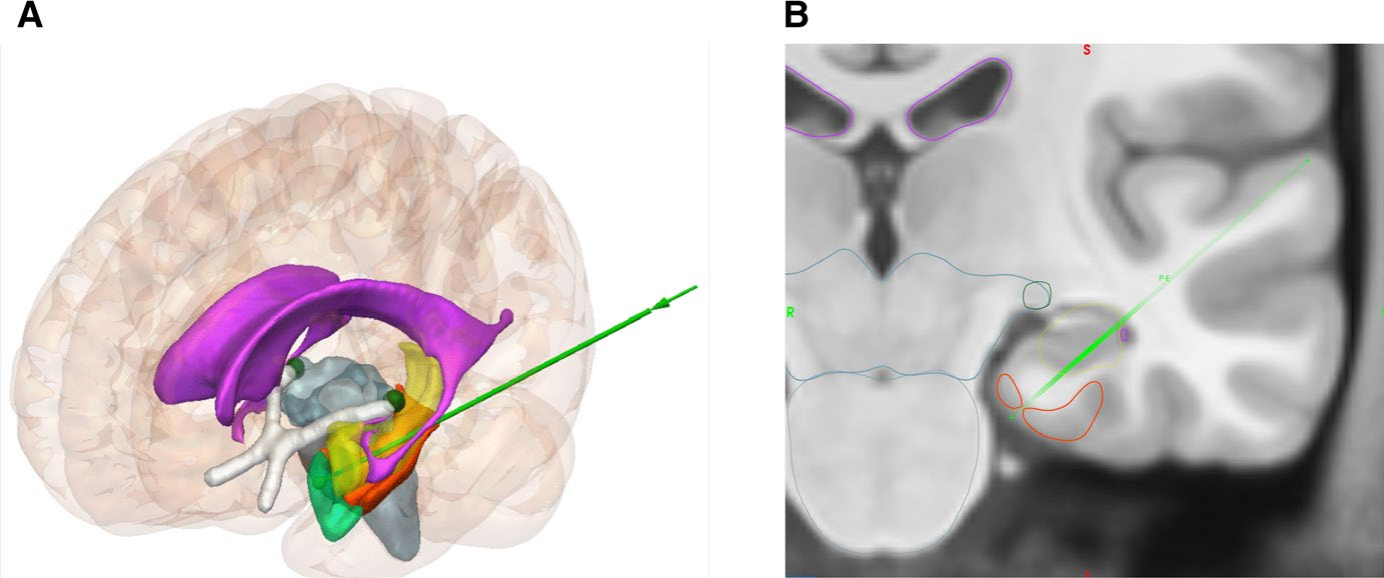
Template‐based A, 3D model and B, coronal MRI of an automated left sided LITT trajectory (light green) at the level of the LGN (dark green) showing its incorporation within the brainstem segmentation (blue). Entire LITT trajectory overlaid onto 2D coronal slice with intrahippocampal (opaque green) and extrahippocampal (transparent green) portions delineating the lateral to medial and superior to inferior angulation of the trajectory. Position of trajectory at level of LGN (green). Amygdalohippocampal complex (yellow), lateral ventricles (pink), parahippocampal gyrus (orange), entorhinal cortex (teal), and optic tract (white) also shown
